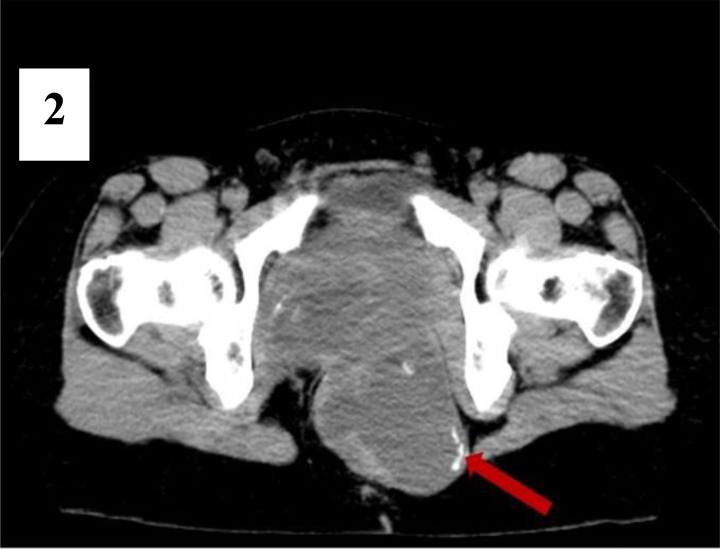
is an axial view of the non-contrast CT scan in which the periphery of the cystic component shows foci of coarse calcification (red arrow).
